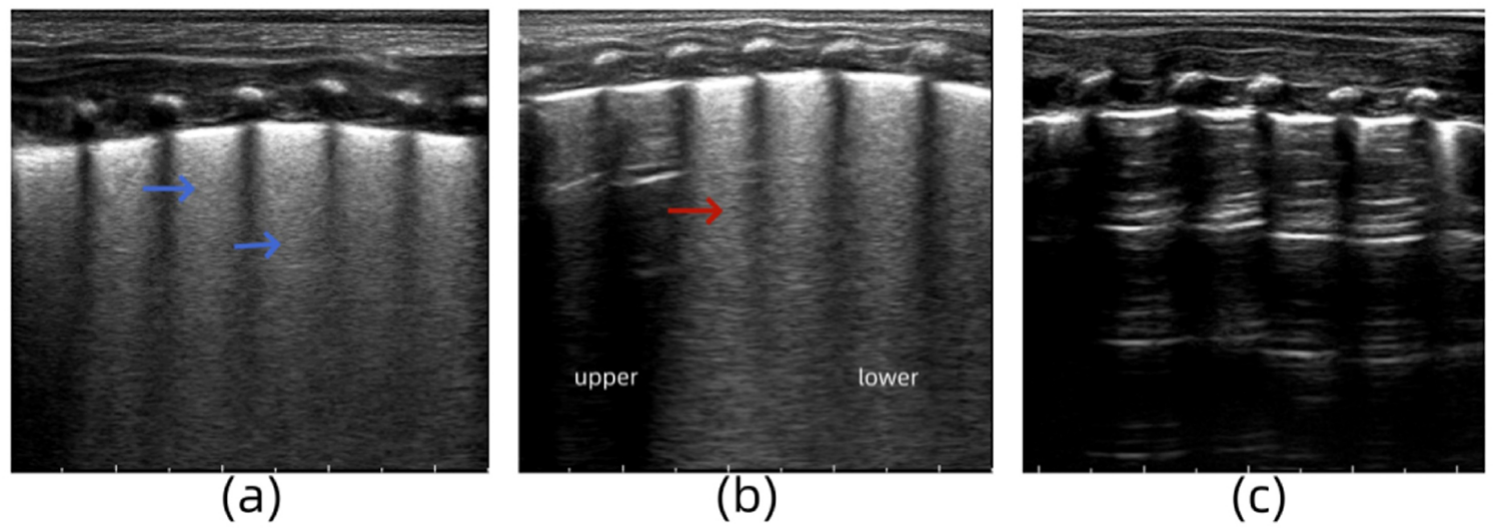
(a) Lung ultrasonography (LUS) examination reveals the disappearance of the pleural line and A-line in both lungs and a “white lung-like” change in both lung fields. (b) LUS re-examination shows “snowflake-like” bronchial inflation in the lungs. (c) LUS findings of both lungs return to normal.
